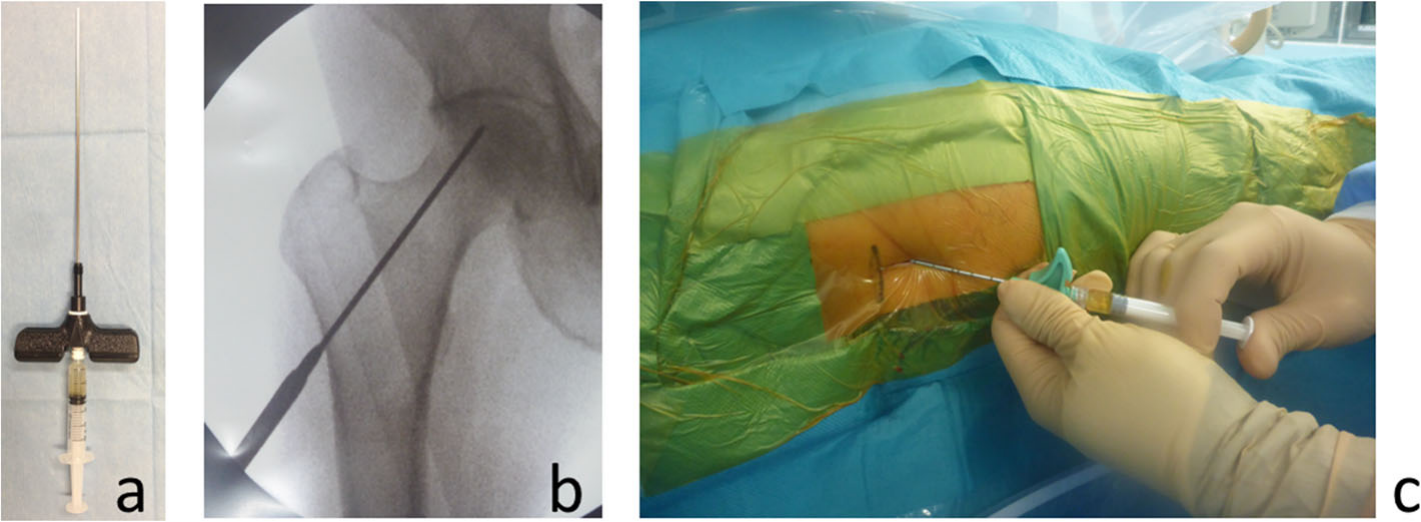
Percutaneous administration of growth factors into the femoral head. Local injection can be percutaneously administered to the target site. This reduces the operating time and damage to surrounding tissue and enables surgery to be minimally invasive. a A syringe with long needle available for percutaneous administration. b An intraoperative radiographic image reaching to the target site. c An intraoperative photograph when pushing the plunger to inject
